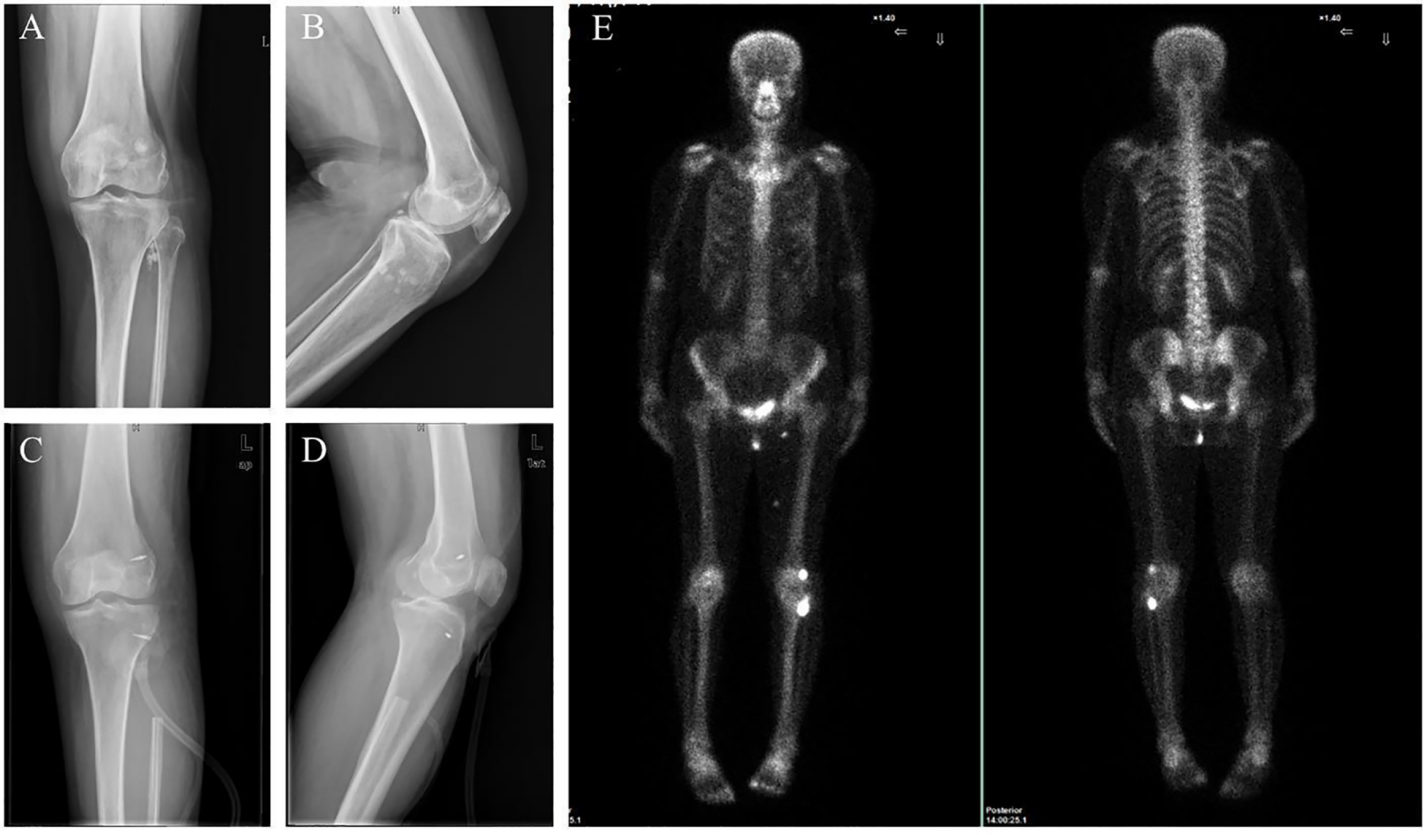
Imaging results indicate that the tumor has recurred. (A, B) X-ray reveals several small, dense nodules near the left knee joint. (C, D) Radiographs of the left knee after surgery. (E) SPECT showed increased bone metabolism in the left knee joint region.
